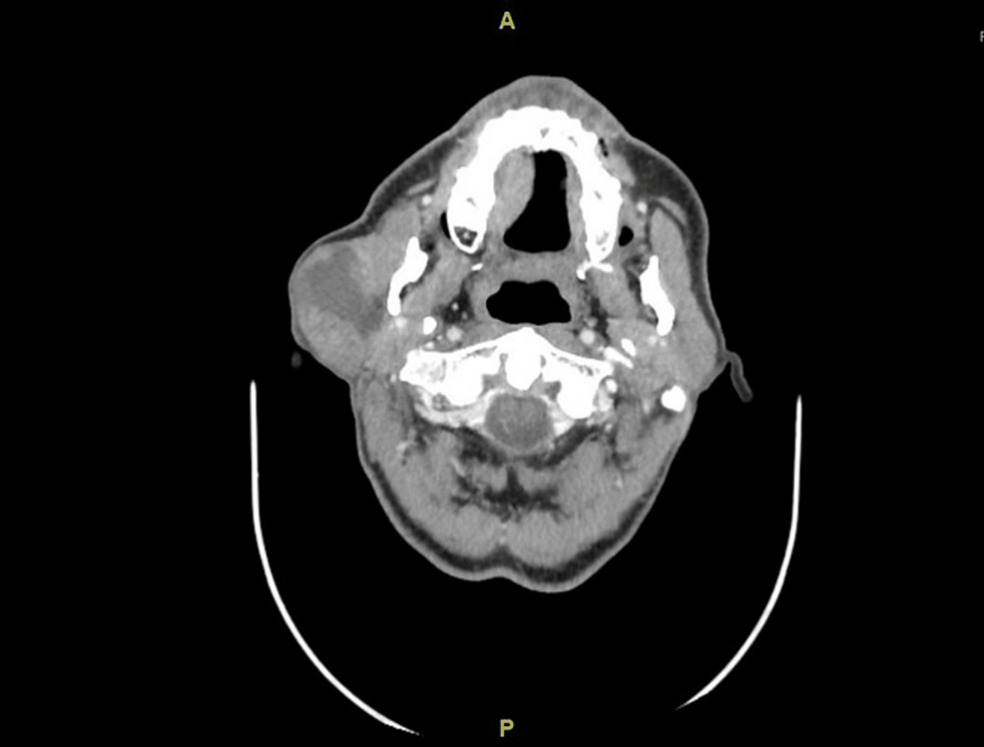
A contrast-enhanced axial CT scan shows a round, well-defined mass in the superficial lobe of right parotid gland with homogeneous enhancement.
